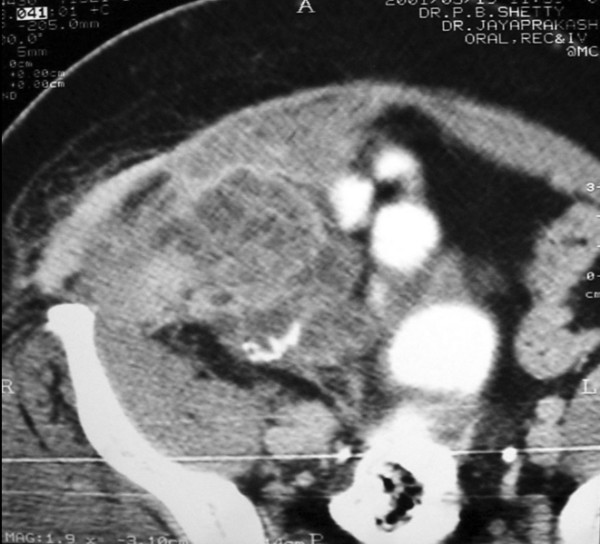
CT scan of the abdomen showing the involvement of abdominal wall muscles and adhesions to neighboring intestines.
